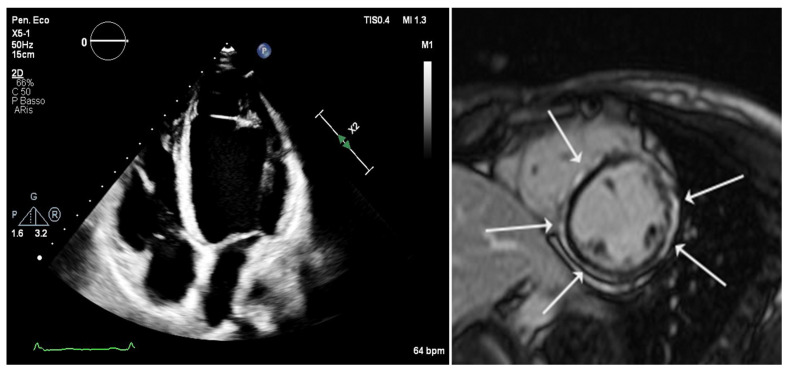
Multimodality cardiac imaging of left-dominant arrhythmogenic cardiomyopathy (LV-ACM) in a patient with a desmoplakin (DSP) variant. Left: Apical four-chamber view from a standard transthoracic echocardiogram demonstrating significant left ventricular (LV) dilation. Right: Corresponding short-axis late gadolinium enhancement (LGE) image from a cardiac magnetic resonance (CMR) scan confirming the underlying tissue pathology, showing a characteristic non-ischemic “ring-like” pattern of replacement fibrosis (hyperenhancement, white arrowheads), primarily localized to the subepicardial layer of the LV wall.
